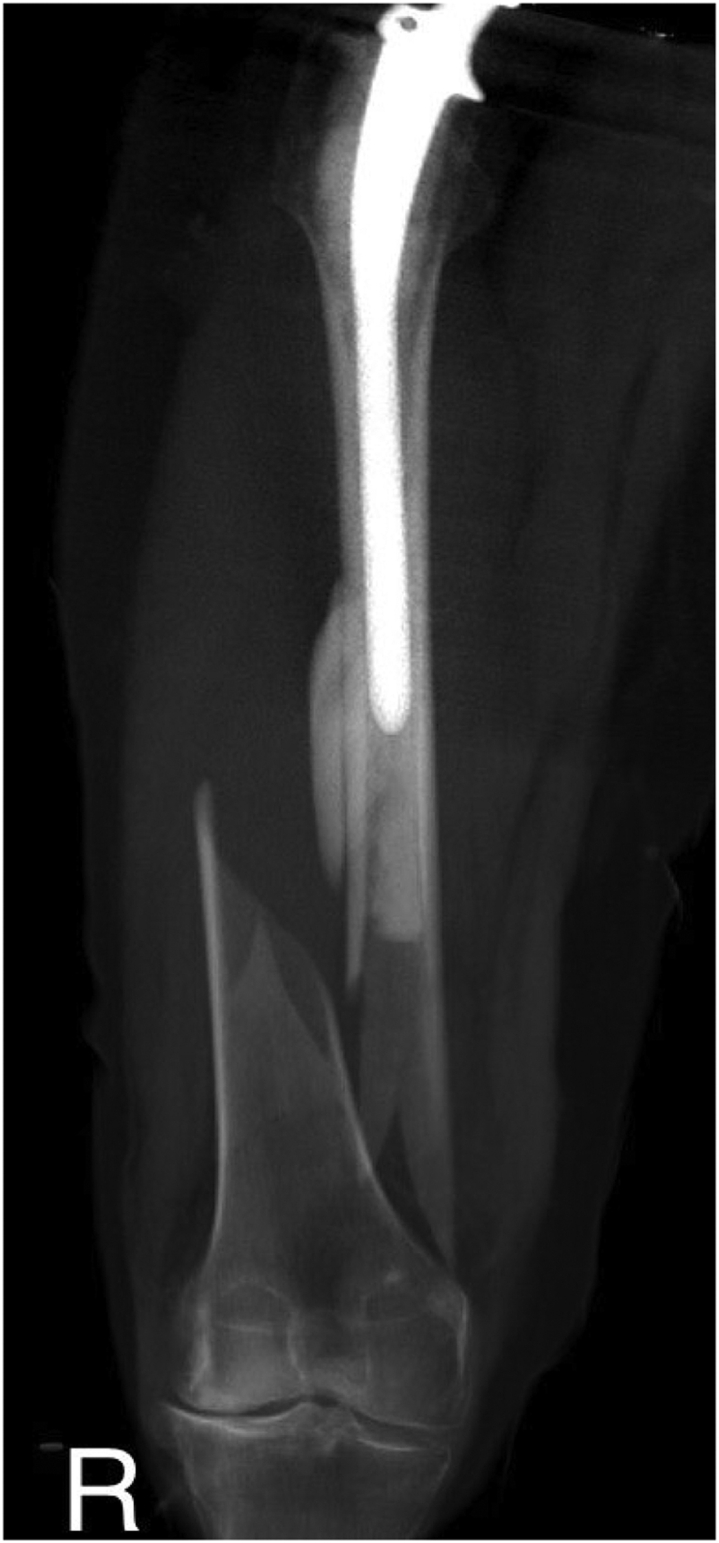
CT scan showing a Vancouver C type diaphyseal fracture of the right femur. The revision hip arthroplasty with a cemented stem remaining intact.
